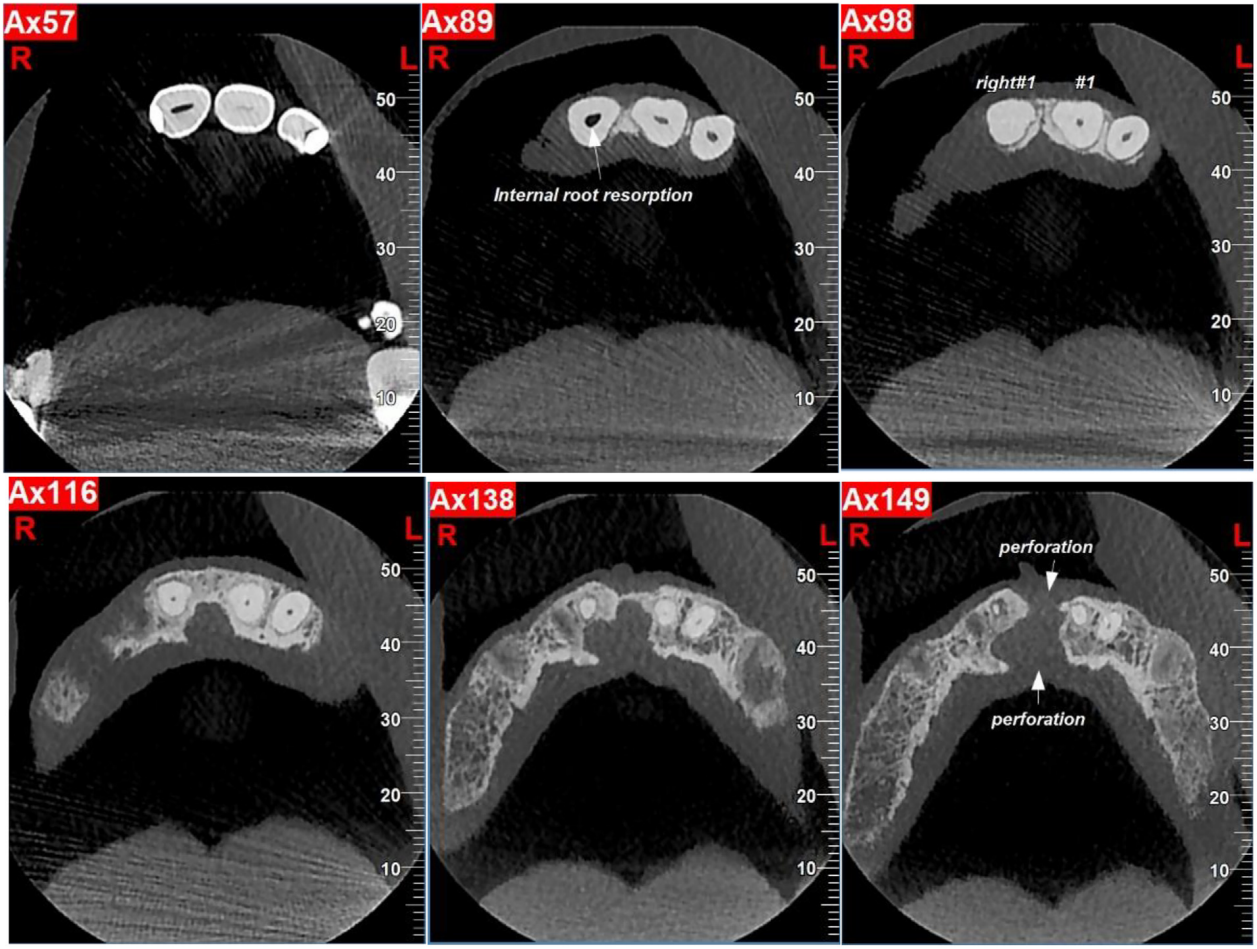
Occlusal views of CBCT images show internal root resorption, bone loss and perforation of cortical plates.
